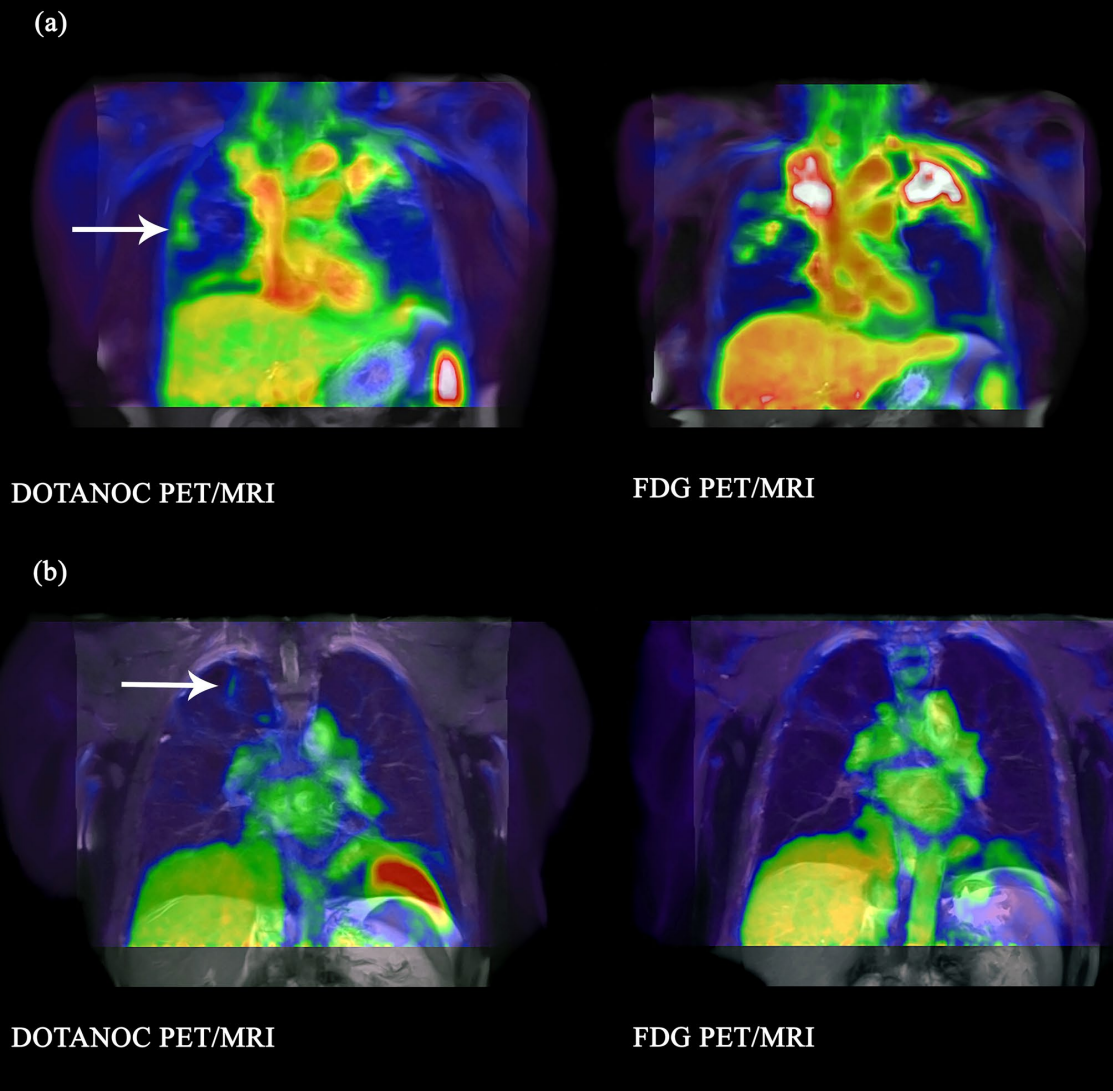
Lesions visualised with DOTANOC but not FDG in pulmonary TB (a) Lesion in right middle lobe visualised with DOTANOC, volume 0.6 cm^3^, SUVmax 1.92. No uptake with FDG. 55-year-old woman, smear 3+, on Day 8 of TB treatment. (b) Lesion at apex right upper lobe visualised with DOTANOC, volume 0.4 cm^3^, SUVmax 1.80. No uptake with FDG. 57-year-old man, smear negative, on Day 11 of TB treatment.
